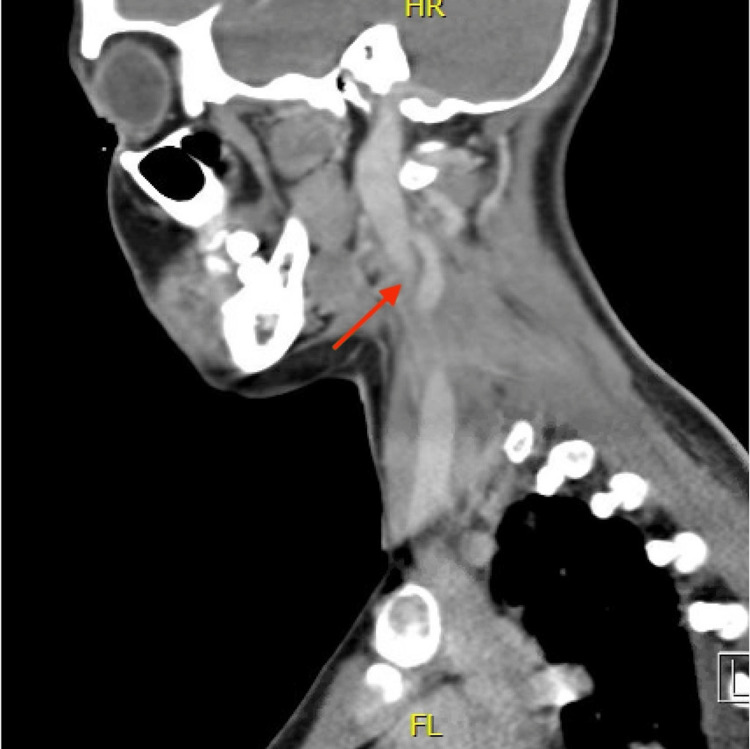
CT head (sagittal) Filling defect consistent with acute thrombophlebitis of the right internal jugular vein extending from C5-6 level to C3 level (red arrow)
